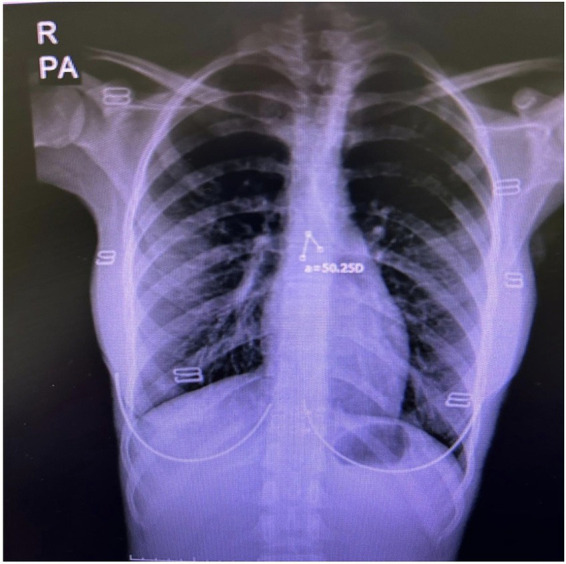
Measurement of the subcarinal angle (SCA) on a posteroanterior chest radiograph (PA-CXR) using the PACS. The subcarinal angle was measured on posteroanterior chest radiographs as the angle formed between the medial borders of the right and left main bronchi at the tracheal bifurcation. Measurements were taken using the digital measurement tools available in the PACS by evaluating the angle between the bronchial axes. Since there is no universally accepted threshold defining a “narrow” SCA in the literature, SCA values were analyzed as a continuous variable in the statistical analysis.
